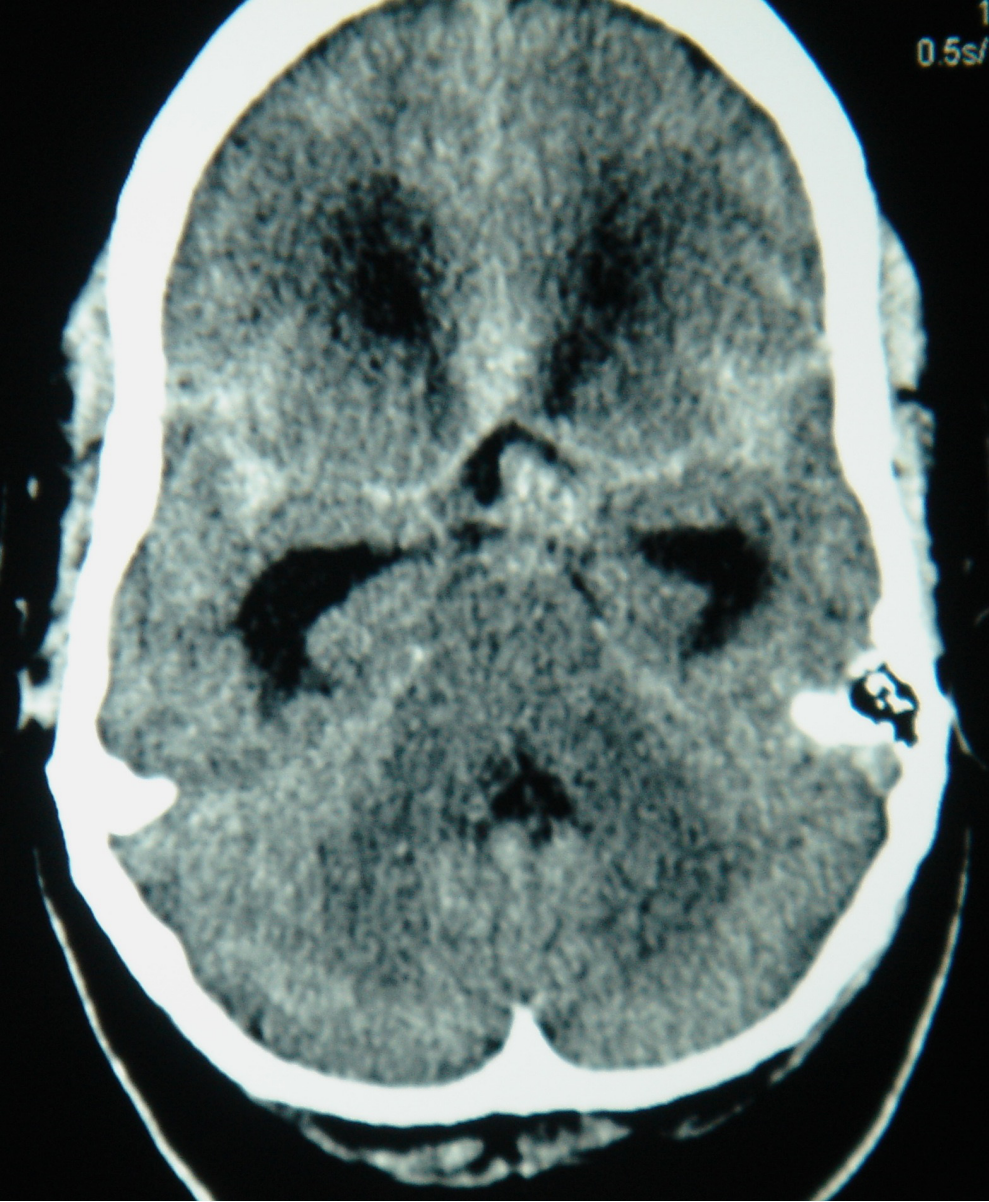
Unenhanced computed tomography scan of her brain showing diffuse subarachnoid hemorrhage in the basal cisterns. Abnormal hyperdense signals in the prepontine cistern indicate an underlying vascular lesion.
